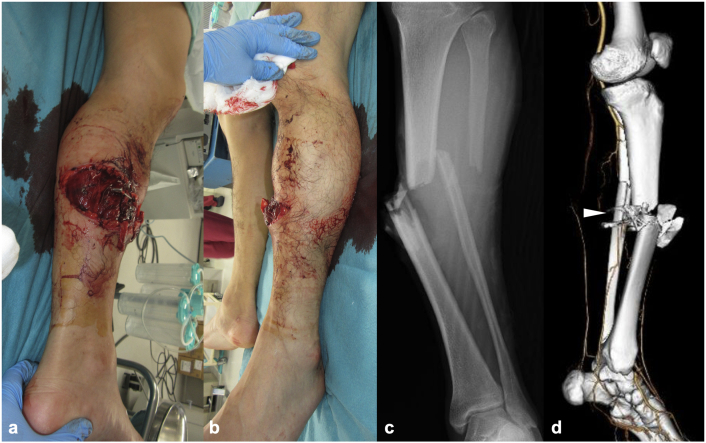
The initial clinical and radiographic findings at presentation of the patient, a 38-year-old male. (a) Medial-posterior view of the left lower leg. (b) Anterolateral view of the left lower leg. (c) Anteroposterior plain radiograph of the left lower leg showing a diaphyseal tibial fracture. (d) Three-dimensional CT angiography of the tibia and surrounding vasculature. The posterior tibial artery was interrupted at the level of the tibial fracture (*(white arrowhead*).
